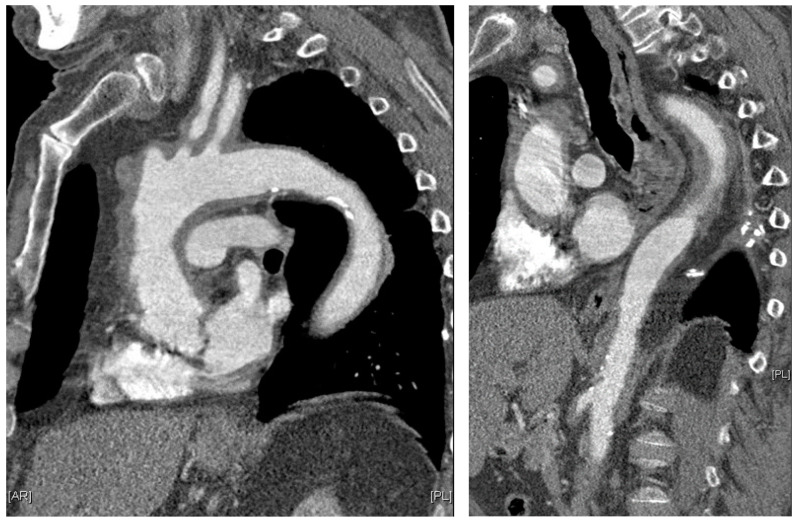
Parasagittal thoracic and sagittal abdominal MPR images on contrast-enhanced computed tomography: pronounced, circular, long-stretch vessel wall thickening of the aorta and its branches in giant cell arteritis. Courtesy of Dr. Corinna Schorn, Rheumatology Center, Rhineland-Palatinate.
